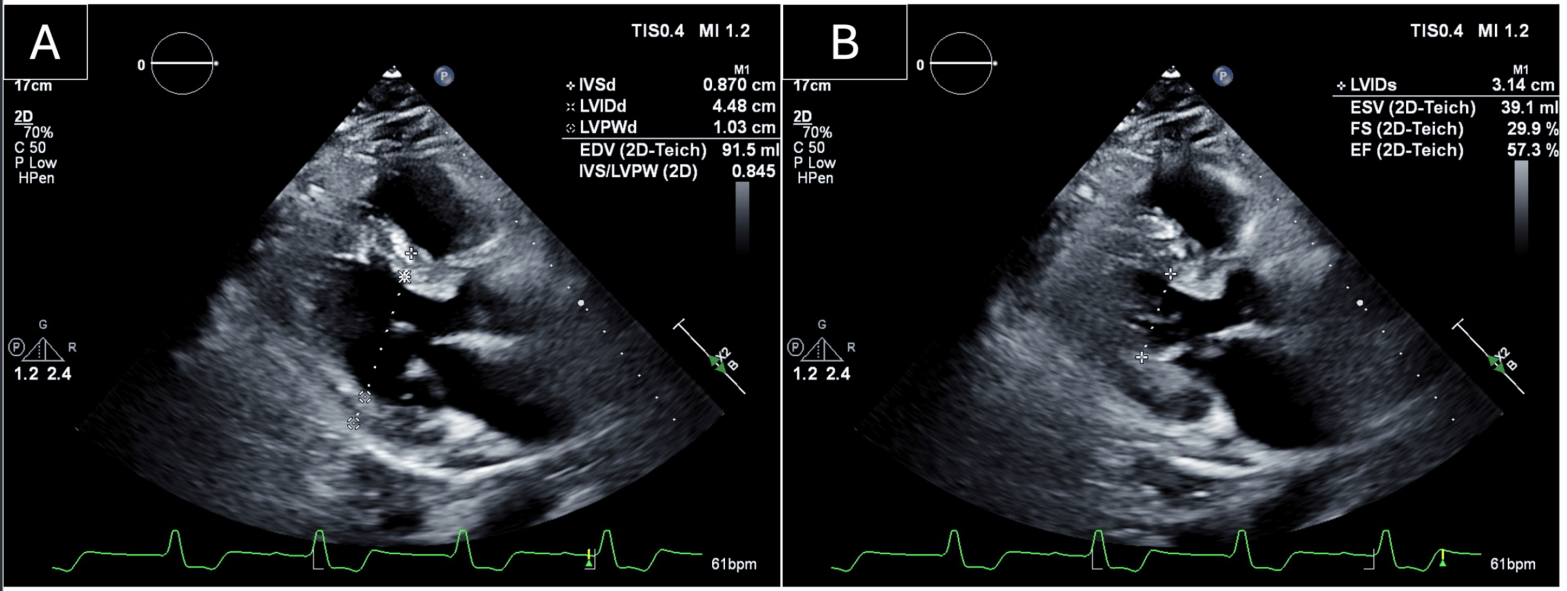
Echocardiographic Views in Immune Checkpoint Inhibitor-Associated Myocarditis (A) Parasternal long‑axis view at end‑diastole, with calipers measuring the left‑ventricular internal diameter in diastole (LVIDd = 4.48 cm); measurement points are shown. (B) Parasternal long‑axis view at end‑systole, demonstrating LVIDs = 3.14 cm; fractional shortening (29.9%) and end‑systolic volume (39.1 mL) were calculated using the Teichholz linear method [10].
